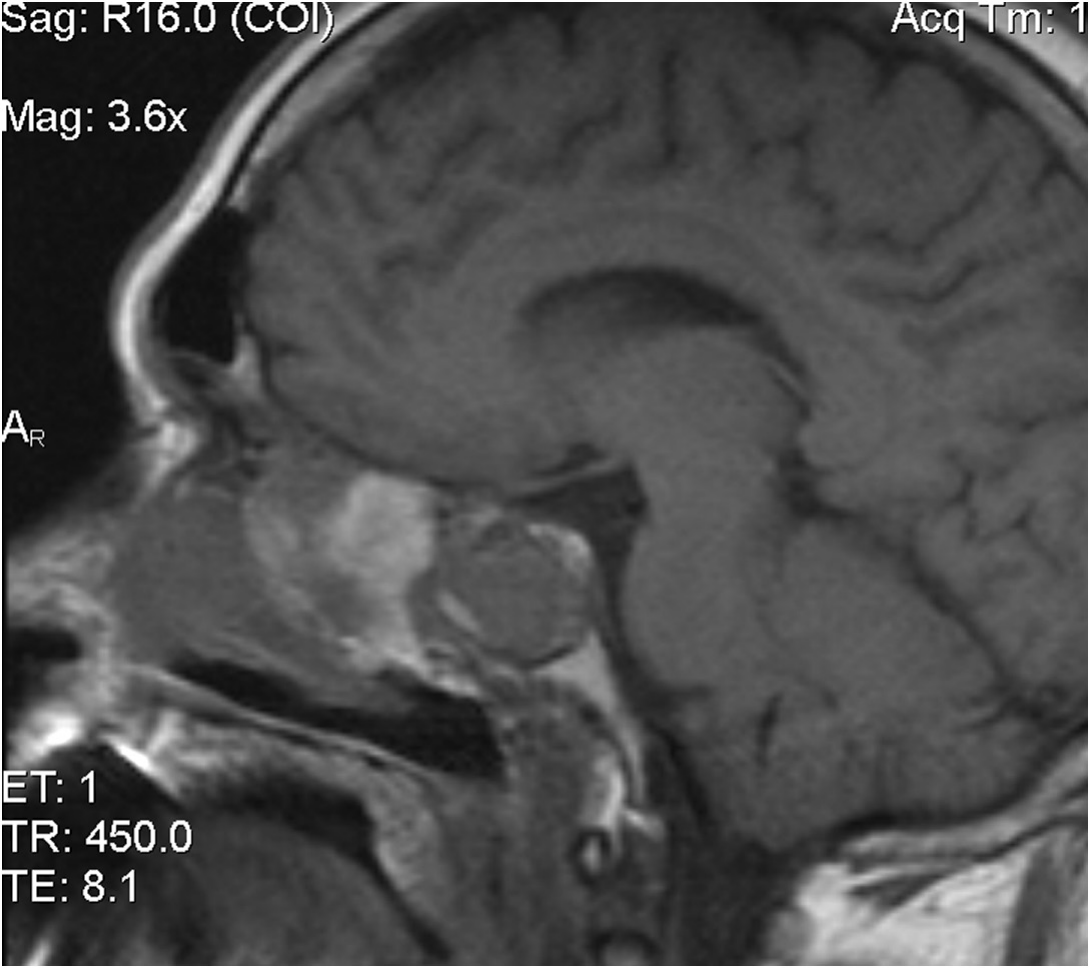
MRI image of mass in sphenoid sinus/optic nerve involvement sagittal
